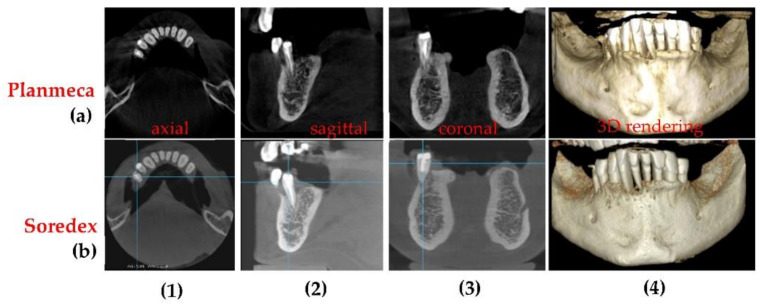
3D CBCT performed on the same patient (B.R., female, 53 years old) with (a) the Planmeca unit and with (b) the Soredex unit. Notations: (1) axial, (2) sagittal, (3) coronal, and (4) 3D rendering.
